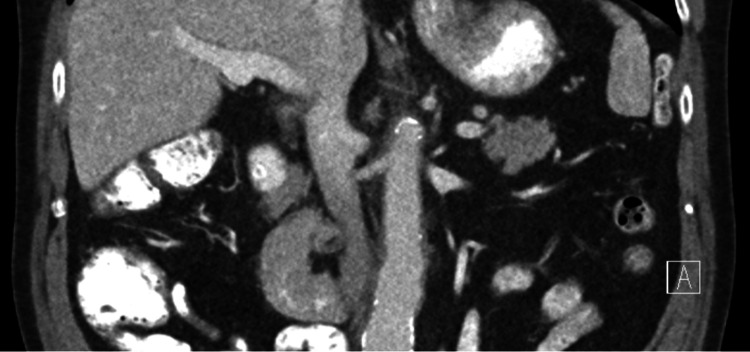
Follow-up CT eight months after therapy initiation Coronal image of follow-up CT eight months after initiation of treatment shows marked interval improvement in the circumferential inflammatory periaortic rind demonstrating improving aortitis CT: computed tomography
